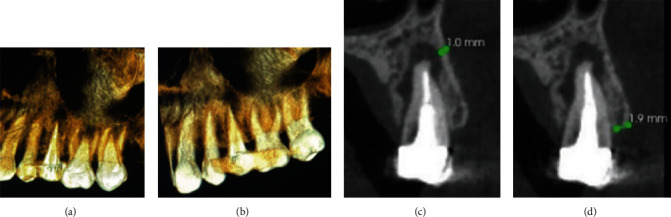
Palatal (a) and facial (b) views of M–D radiographic sections and facial plate thicknesses (c and d).
